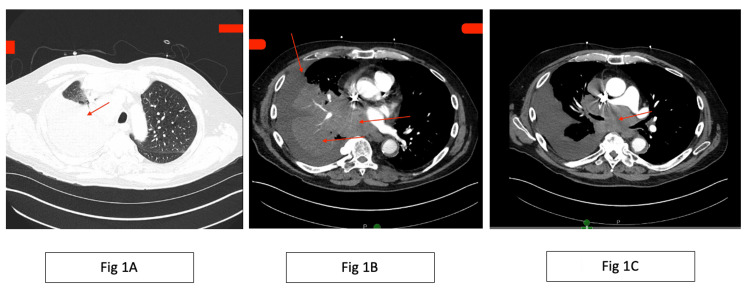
CT scan presentation on day one. Three CT scan images, including lung and soft-tissue windows, showing right-sided pleural effusion in Panel A, mediastinal/hilar adenopathy with collapsed lung and suspicious mass in Panels B and C.
